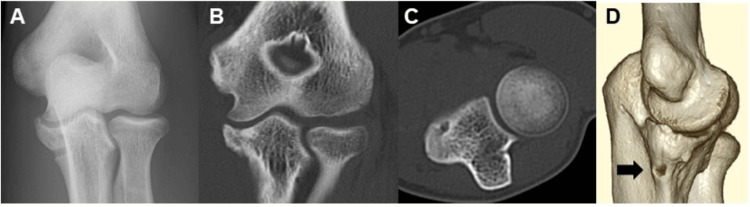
Postoperative radiographic and CT findings of case 2 A. Postoperative anteroposterior radiograph showing anatomical realignment of the fragment following suture bridge fixation. B. Coronal CT image. C. Axial CT image. D. Three-dimensional CT reconstruction demonstrating successful bone union and the position of the lateral knotless anchor used in the suture bridge construct (black arrow).
